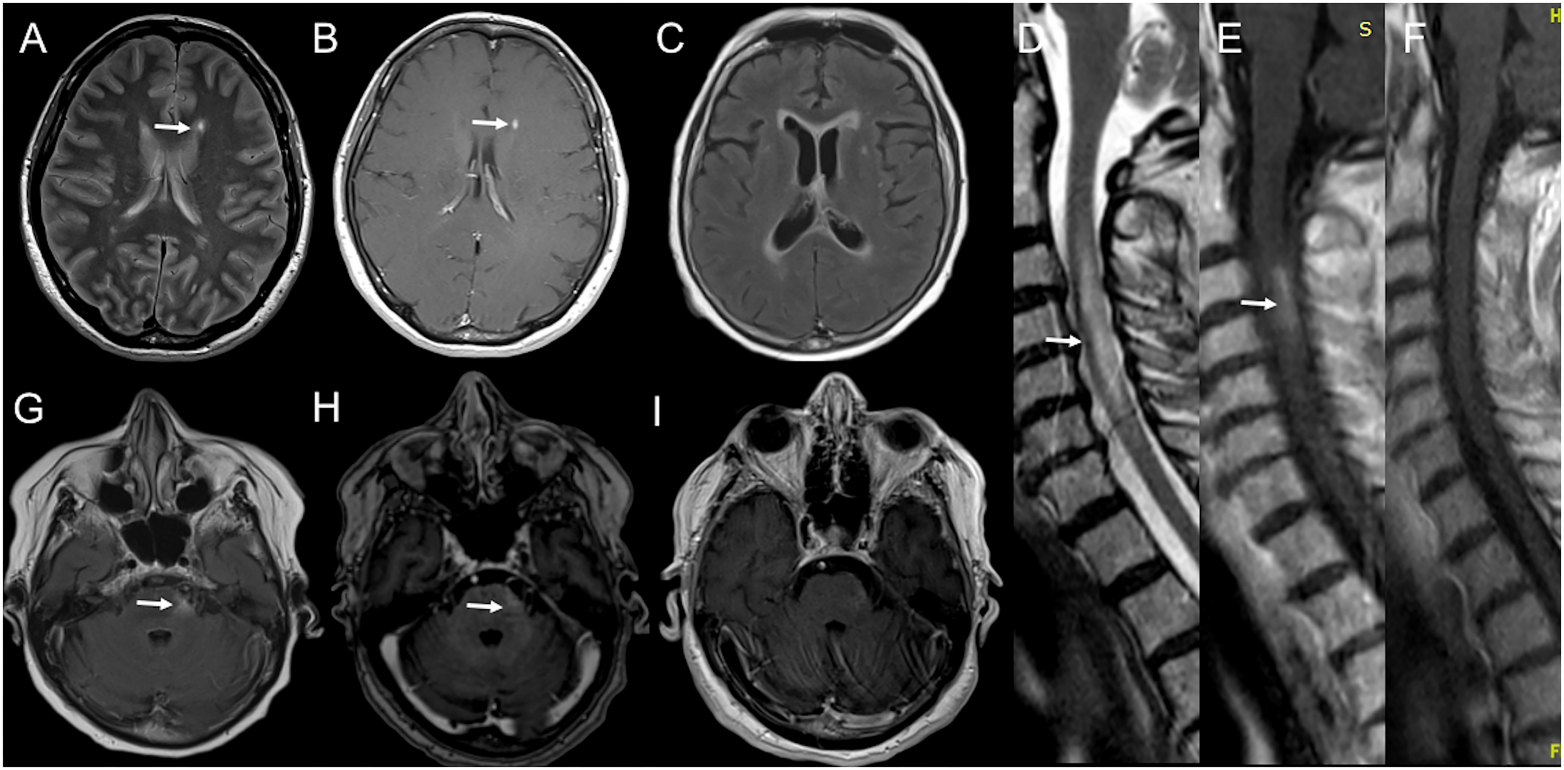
Serial brain and spinal cord MRI scans in Case 5 (A,B) and Case 6 (C–I). Case 5: (A) Brain MRI performed 28 days after BNT162b2 first dose, showing a new left lateral periventricular T2-weighted hyperintensity (arrow) with contrast enhancement [(B); arrow]. Case 6: (D) Spinal cord MRI obtained 12 days after BNT162b2 second dose, showing a T2-weighted hyperintensity from C2 to C7 with swelling (arrow) and gadolinium enhancement [(E); arrow]. (G) Brain MRI performed few days later showed a T2-weighted hyperintensity in left lateral pons (arrow) with blurred contrast enhancement [(H); arrow] and confluent supratentorial bilateral periventricular hyperintensities on FLAIR images (C). (I) 7-days MRI controls revealed the complete resolution of gadolinium enhancement of the left pontine lesion and the volume shrinkage of the cervical lesion with no more contrast enhancement on T1-weighted images (F).
